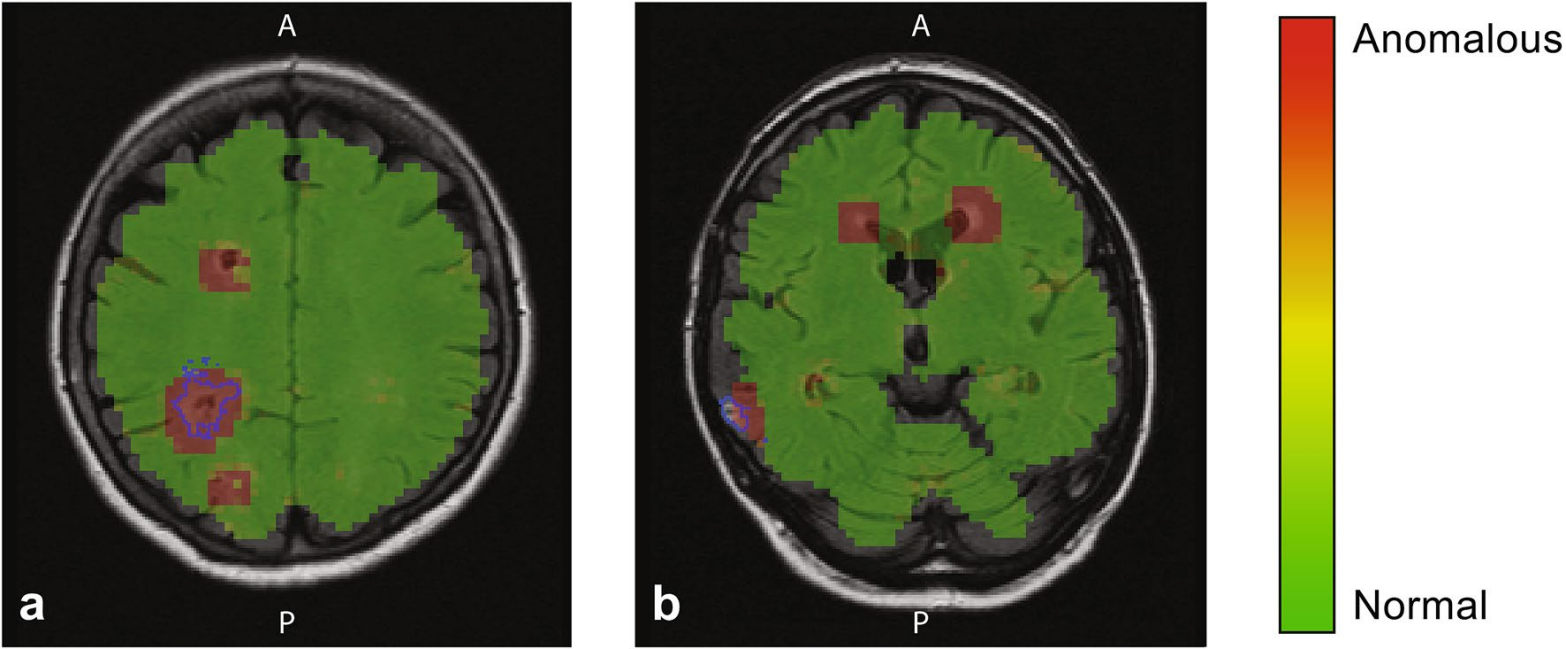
Anomaly score overlay maps on transversal image slices (T2-FLAIR images). A red color indicates an anomaly score ≥ 3, an anomalous location, where green and yellow an anomaly score < 3, normal tissue. The manually annotated brain infarcts are given by the blue outlines. An unannotated brain infarct located in a cranial slice can be observed in (a), anterior (A) of the annotated brain infarct. The anomaly at the posterior (P) side of the brain infarct in (a) is only delineated in a subsequent image slice. (b) Detected cortical brain infarct, and two other suspected anomalous locations, caused by white matter hyperintensities at the horn of the ventricles.
